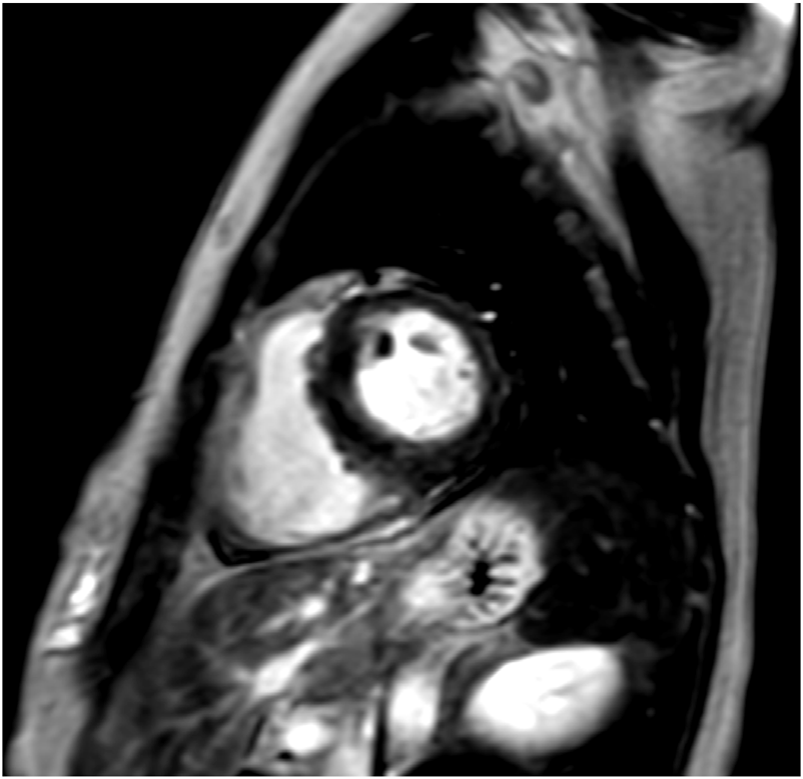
Cardiac magnetic resonance imaging (MRI) of case 1. Cardiac MRI showing no late gadolinium enhancement (LGE).
